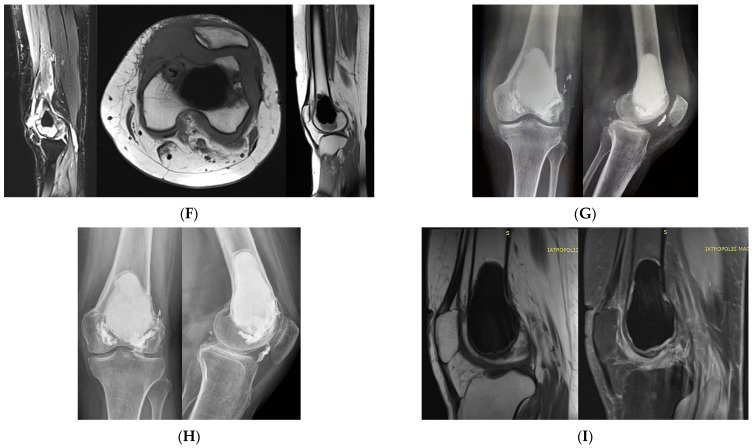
A 49-year-old female patient with giant cell tumor of the distal femur. (A) X-ray image captured at presentation. (B) Magnetic resonance imaging (MRI) at presentation. (C) X-ray image captured after 4 months (7 doses) of preoperative treatment with denosumab. (D) X-ray image captured immediately after curettage and cement filling. (E,F) Local recurrence was observed 2 years after curettage. (G) X-ray image captured immediately after radiofrequency ablation and cementoplasty. (H,I) There was no local recurrence observed at 4 years after radiofrequency ablation and cementoplasty. The patient did not have knee pain.
